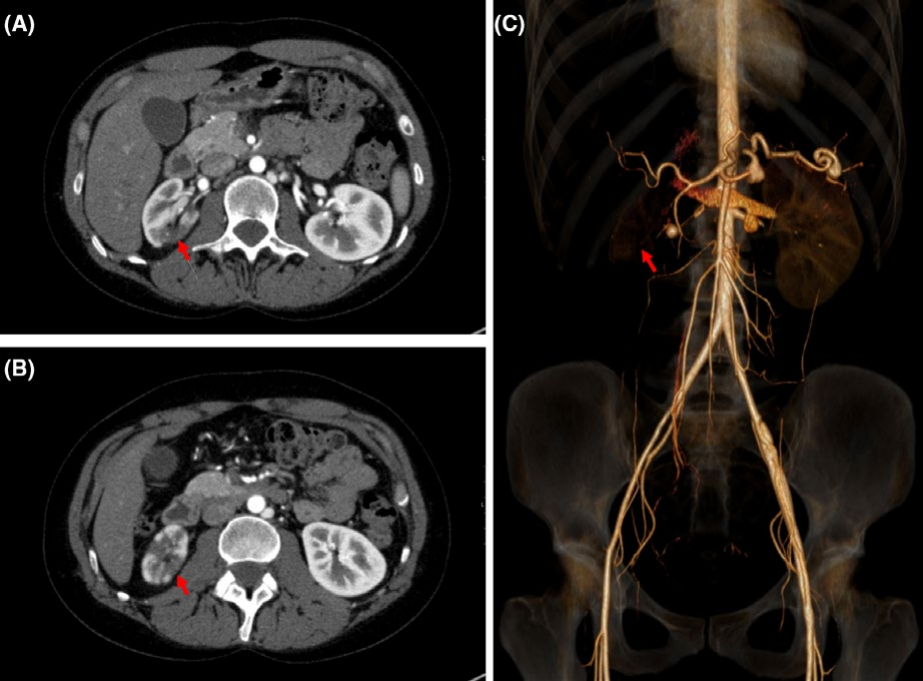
Computed tomography angiogram (CTA) scan showing diffuse cortically based, hypodense areas with nearly no perfusion of the renal parenchyma in the posterior aspect of the upper and lower pole (Panel A and B); 3‐dimensional reconstruction of the CTA demonstrated similar findings (Panel C)
